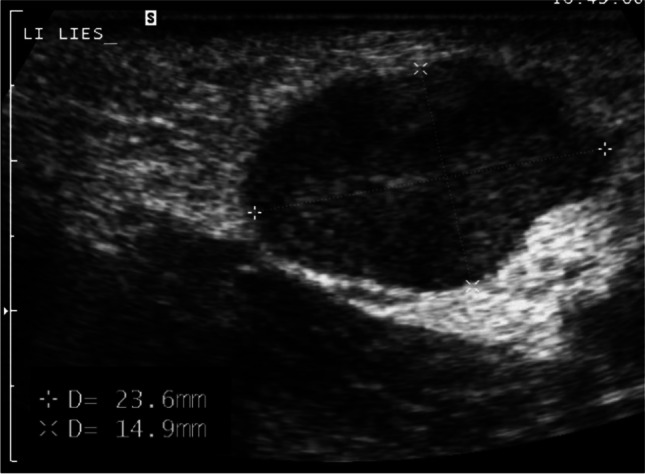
A longitudinal ultrasound image of the left groin a 14-year-old girl (patient 2) shows an enlarged lymph node. Note absence of the normal fatty centre
